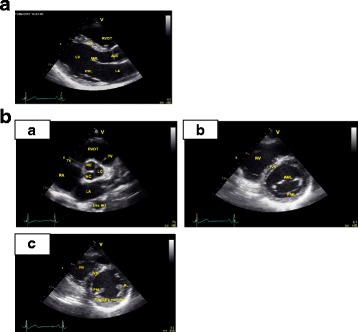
a Parasternal long axis view and main anatomic points. b Parasternal short axis view at three levels. *a* Aortic level. *b* Mitral level. *c* Mild papillary level
